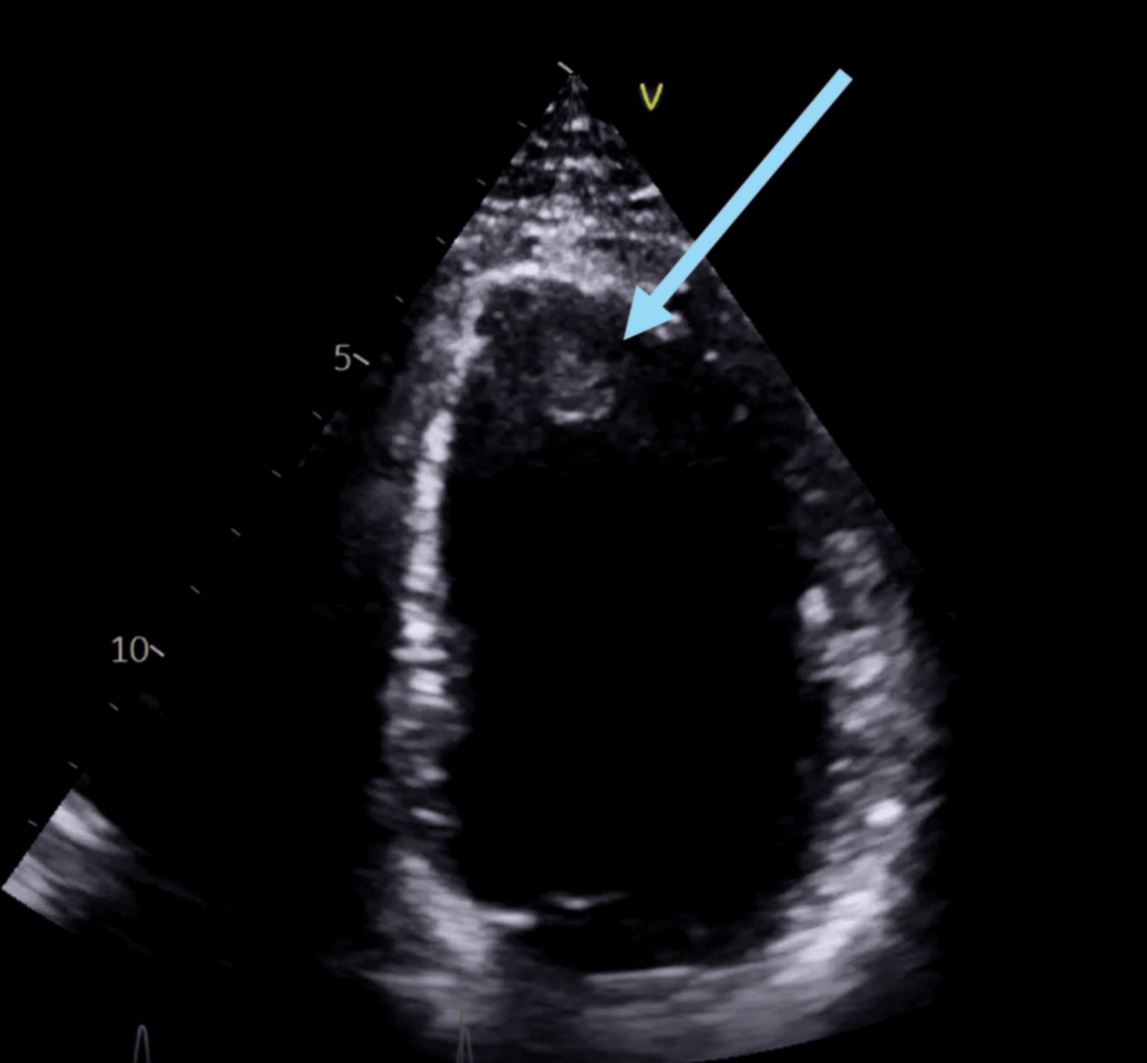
Transthoracic echocardiogram demonstrating a thrombus at the left ventricular apex (highlighted by blue arrow)
